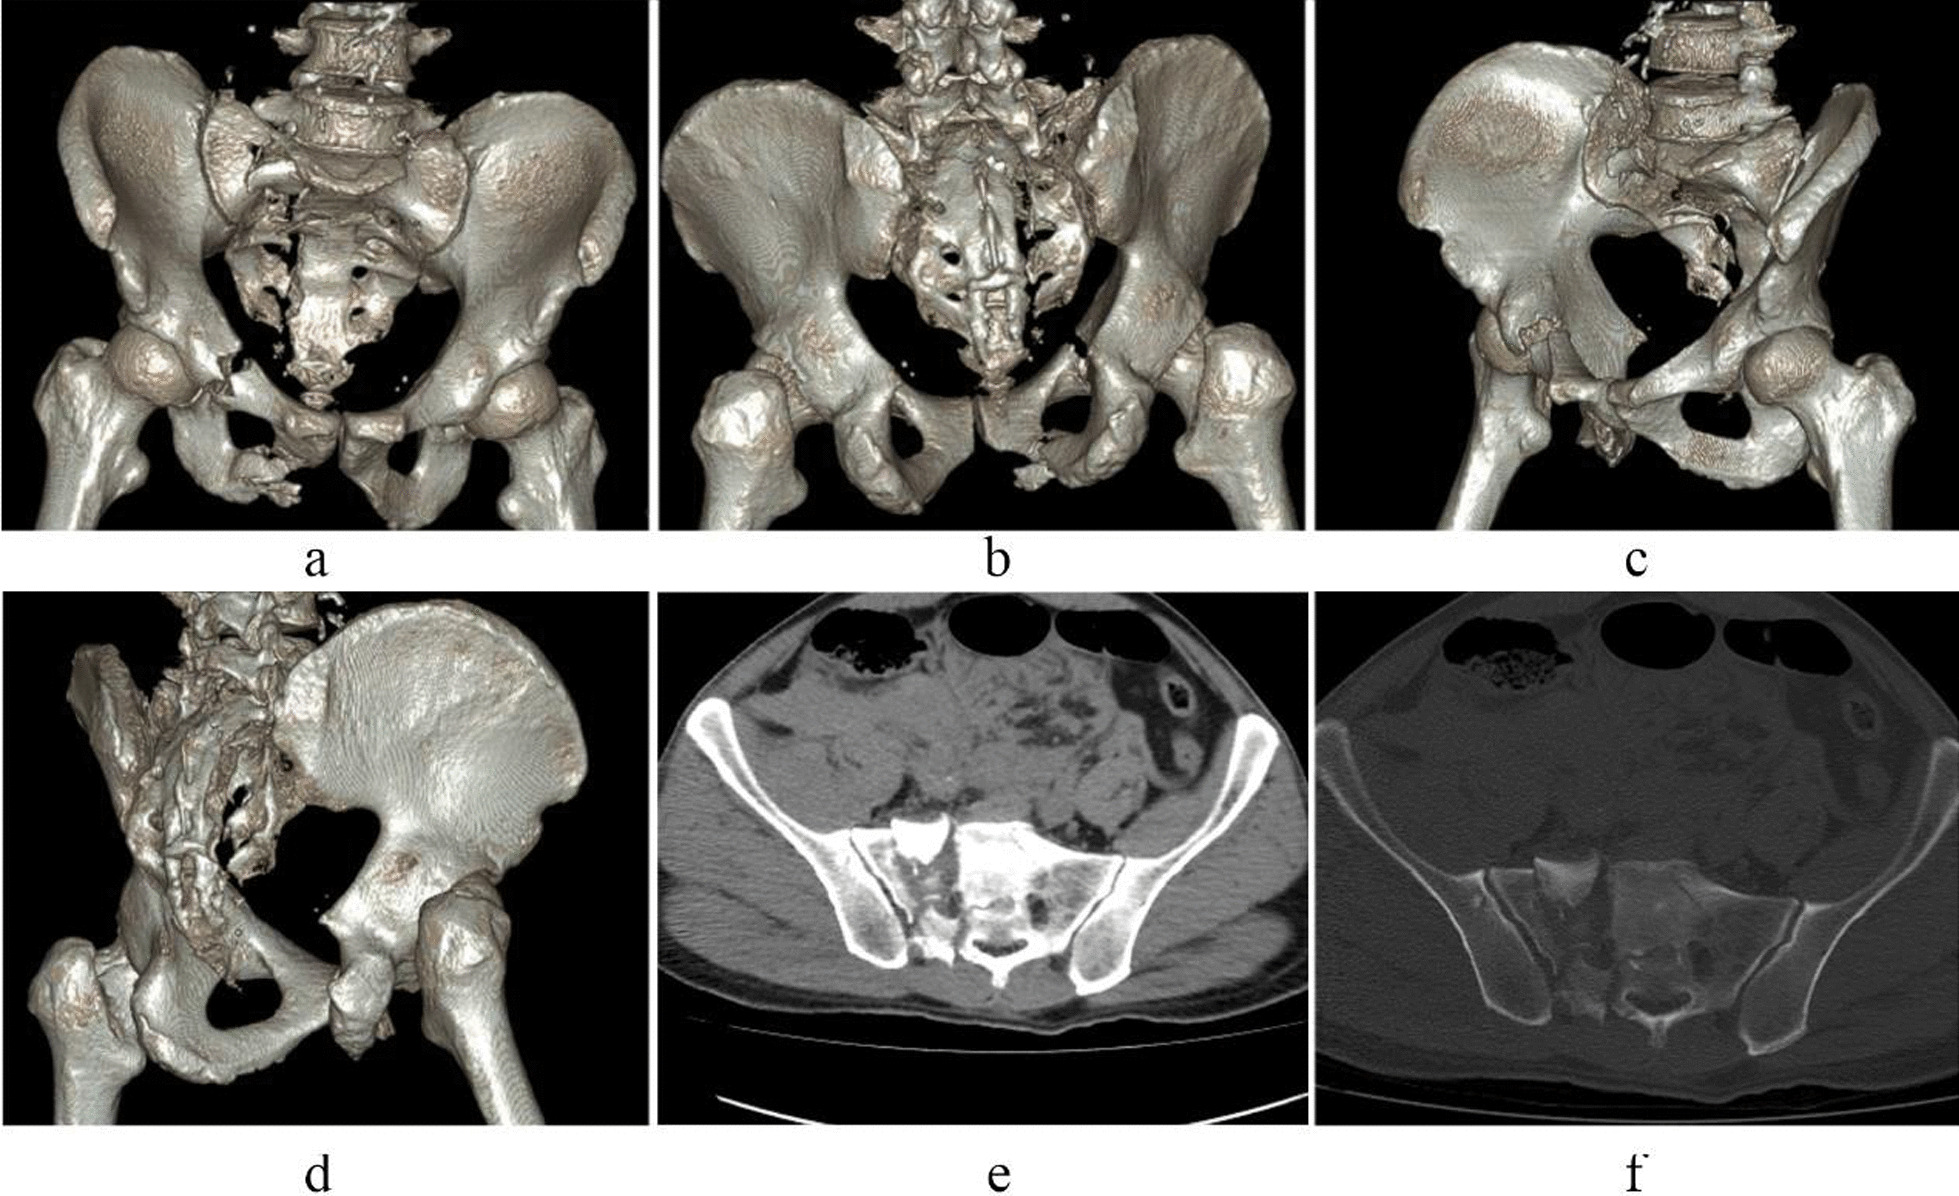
Preoperative CT images of case 4. (a–d CT 3D reconstruction images, e, f CT horizontal slice images.)
